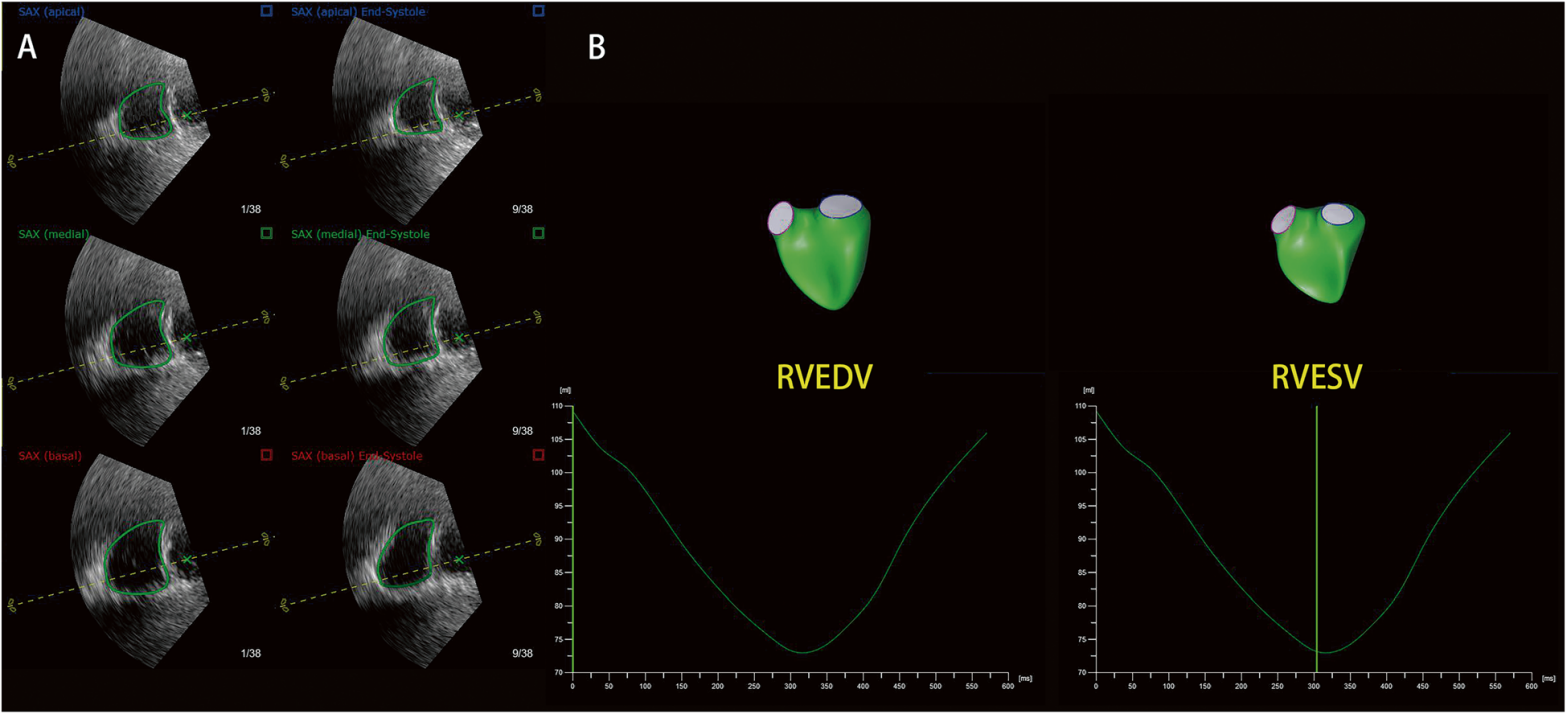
Measurement of 3D Rvol EROA. a RV was traced by dedicated software tools. b A pulsed-wave Doppler sample volume was placed into the RVOT to measure the VTI of outflow velocities. c Using the software to calculate RV volume from the 3D TTE data set, SV_RV_ was calculated by subtracting RVESV from RVEDV
